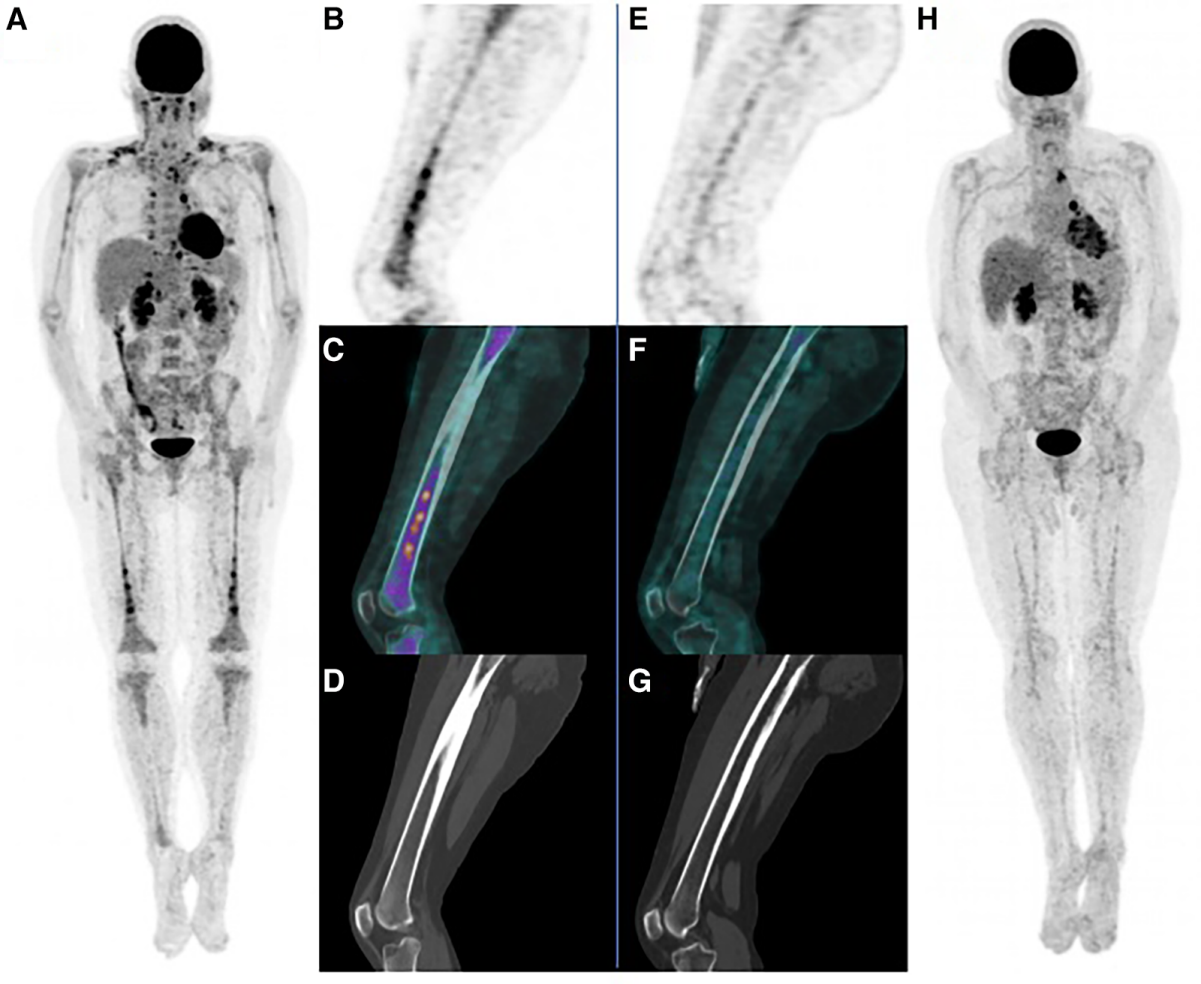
First PET scan with maximal intensity projection (MIP) (A), sagittal PET (B), fusion (C), and CT (D). Final post treatment PET scan with MIP (H), sagittal PET (E), fusion (F), and CT (G), showing completely reduced signal of bone lesions, and almost complete peri-renal collection response.
